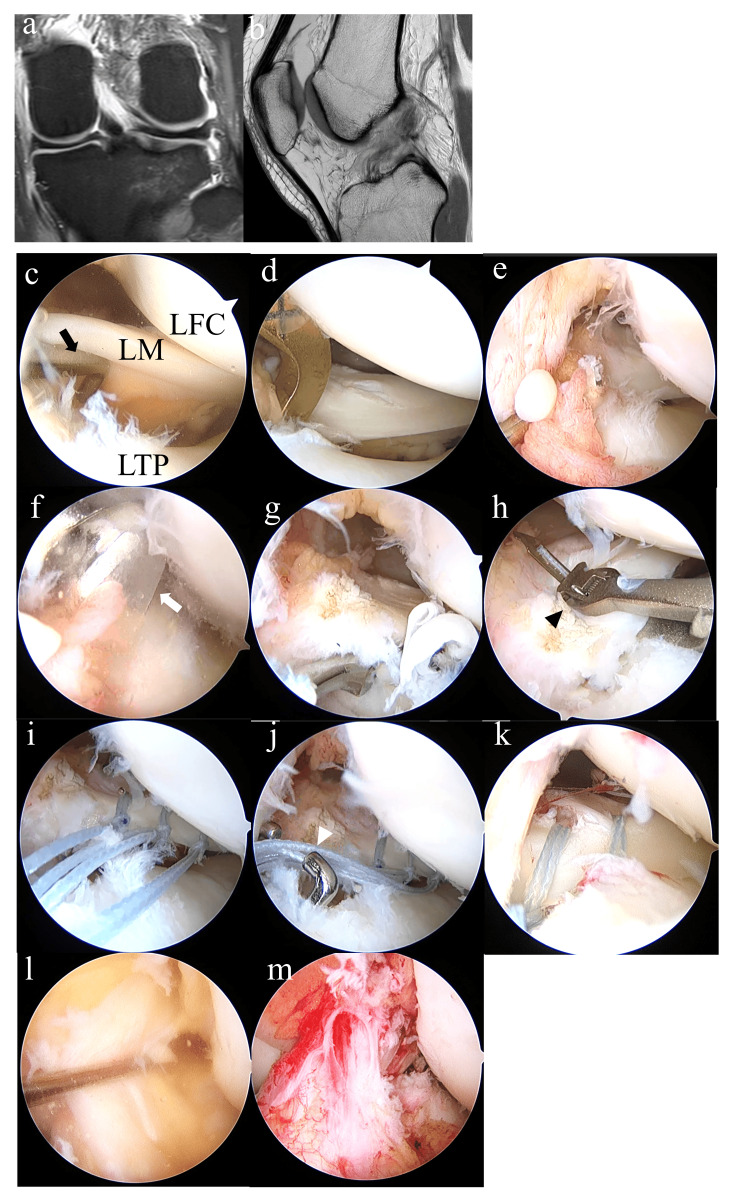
Representative case 1 (a, b) Preoperative MR images. (a) No apparent meniscus injury. (b) Torn ACL. (c-m) Arthroscopic findings. (c) Type 2a LMPRT with positive lift-off sign is observed by probing (arrow). (d) The tibial ACL guide is set at the anatomical attachment site of the LM posterior root. (e) A 2.4-mm guide wire is inserted. (f) The bone tunnel is created with a 6.0-mm cannulated reamer (white arrow). (g) Articular cartilage around the bone tunnel is removed with a curette until subchondral bone is exposed to promote adhesion of the meniscus. (h) A 2-0 strong suture for racking hitch knot is placed in the meniscus using Knee Scorpion Suture Passer (arrowhead). (i) Three racking hitch knot sutures securely hold the torn edge of the meniscus. (j) The sutures are introduced into the tunnel using a suture retriever (arrowhead). (k) After fixation, reattachment of the LM posterior root to the insertion site is confirmed. (l) Torn ACL. (m) Reconstructed ACL with preserved remnant.
